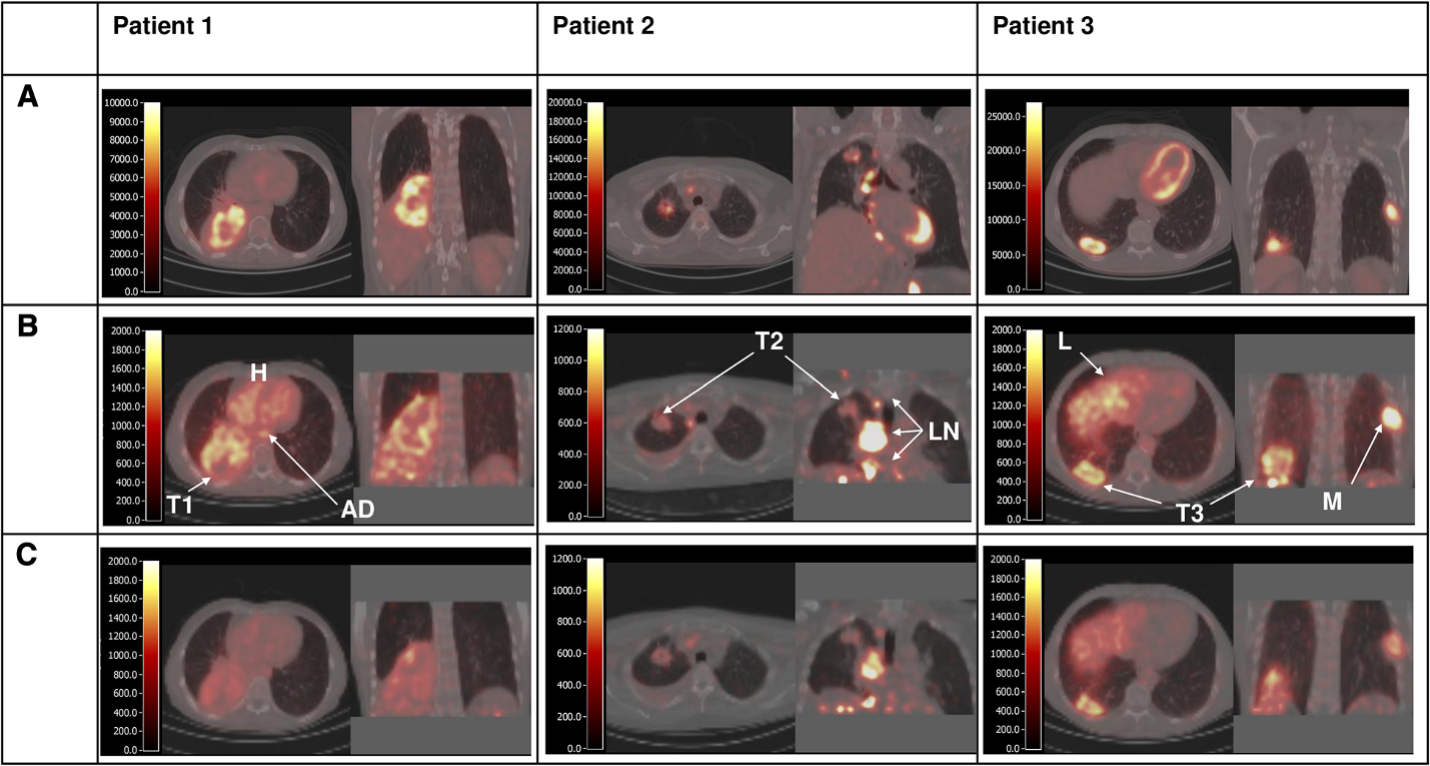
Fused PET/CT images using FDG (A) and^89^Zr-bevacizumab at day 4 (B) and day 7 (C). Per scan, an axial and coronal slice is shown. The color scale, indicating Becquerels per milliliter (BQML), ranges from 0 to a maximum value that corresponds with a SUV value of 6. In patient 1, a large tumor in the right lower lobe is seen. There is increased FDG uptake in the outer rims of the tumor and reduced uptake in the center of the tumor (probably due to necrosis). The ^89^Zr-bevacizumab image on day 4 also shows high uptake in the outer rims of the tumor (T1) and high blood activity concentration (aorta descendens (AD) and heart chambers (H)). Low uptake is found in non-tumor tissues, such as healthy lung, fat, and muscle. The ^89^Zr-bevacizumab image on day 7 shows high uptake in the outer rims of the tumor but low uptake in healthy tissues as well as low blood activity concentrations. In patient 2, the FDG scan shows increased uptake in both the primary tumor in the right upper lobe and enlarged mediastinal lymph node metastases. Interestingly, the ^89^Zr-bevacizumab images only show increased uptake in the lymph node metastases (LN), while the uptake in the primary tumor (T2) on both days 4 and 7 is faint. In patient 3, the primary tumor, the mediastinal lymph node metastases, and rib metastasis all show increased FDG uptake. The ^89^Zr-bevacizumab scans on days 4 and 7 show the highest uptake in the rib metastasis (M) and the primary tumor (T3), and moderate to high uptake is seen in the liver (L).
